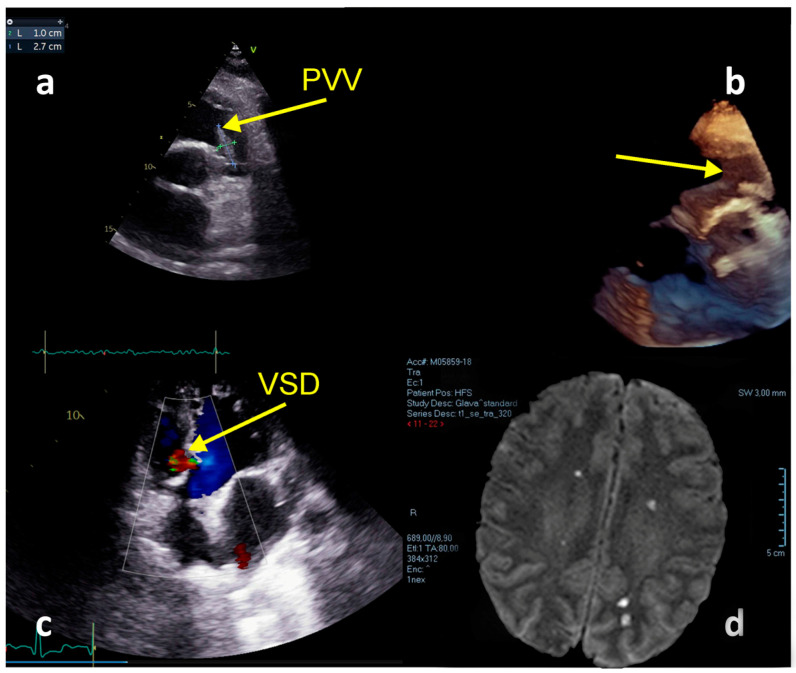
Echocardiography and MRI imaging—Case 2. (a) Floating vegetation in the RVOT. (b) TTE + 3D reconstruction. (c) Residual VSD; (d) MRI—Multiple septic brain embolization.
